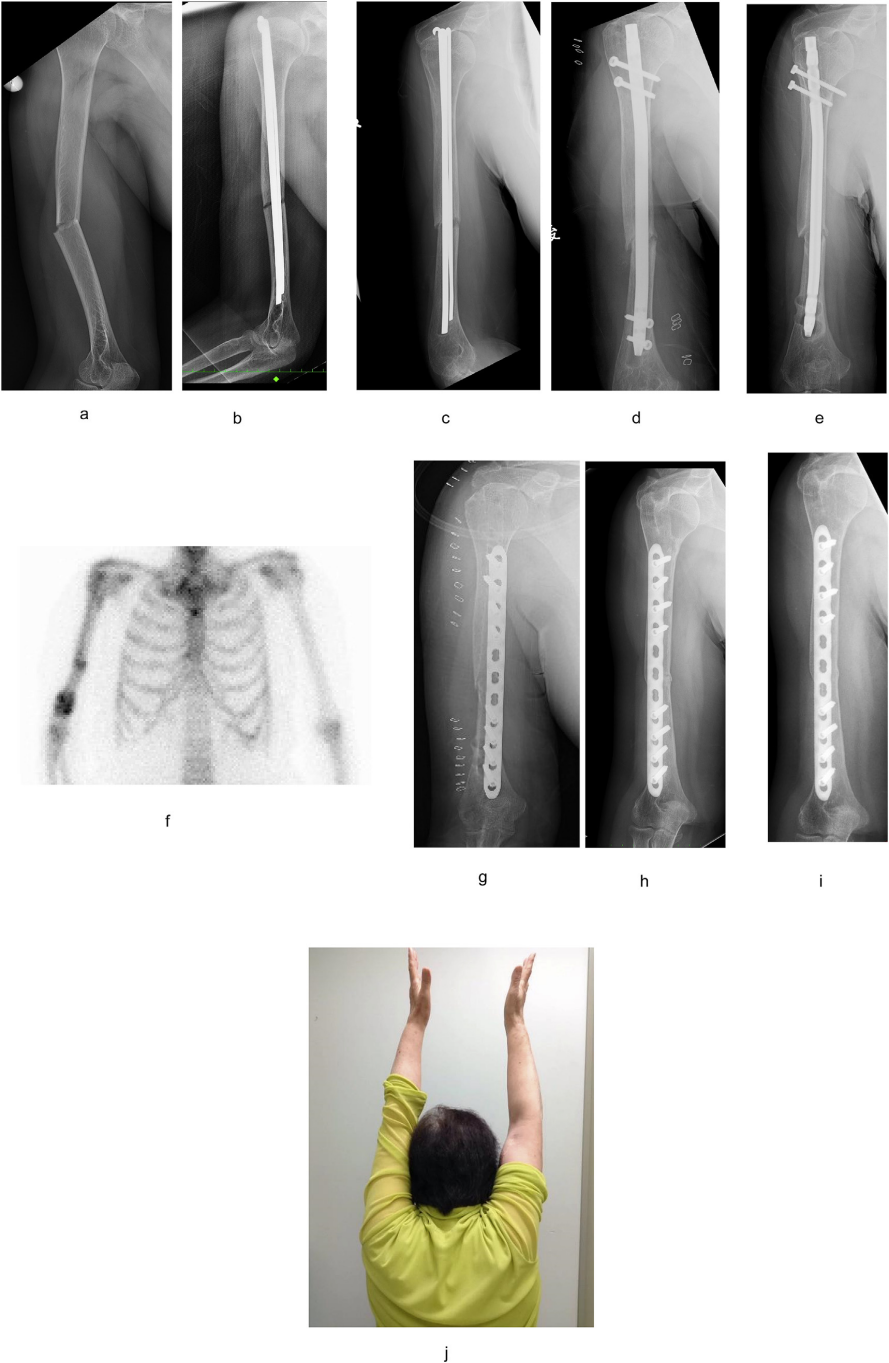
Views of Case 2. (2a) AO classification: 12A3 fracture. (2b) Just after the 1st surgery with RUSH pin. (2c) Five months after the 1st surgery. Diagnosed as nonunion. (2d) Exchange nailing was performed. (2e) One year after the 2nd surgery. Little callus formation was observed around nonunion area. (2f) Bone scintigraphy showed an increased accumulation in the place of nonunion. (2g) We performed the reoperation using the MIPO method without autogenous bone grafting. (2h) One year after the surgery, bony union was obtained. (2i) Final follow-up 2 years after the surgery. (2j) She obtained full range of motion with no restrictions in activities of daily life.
